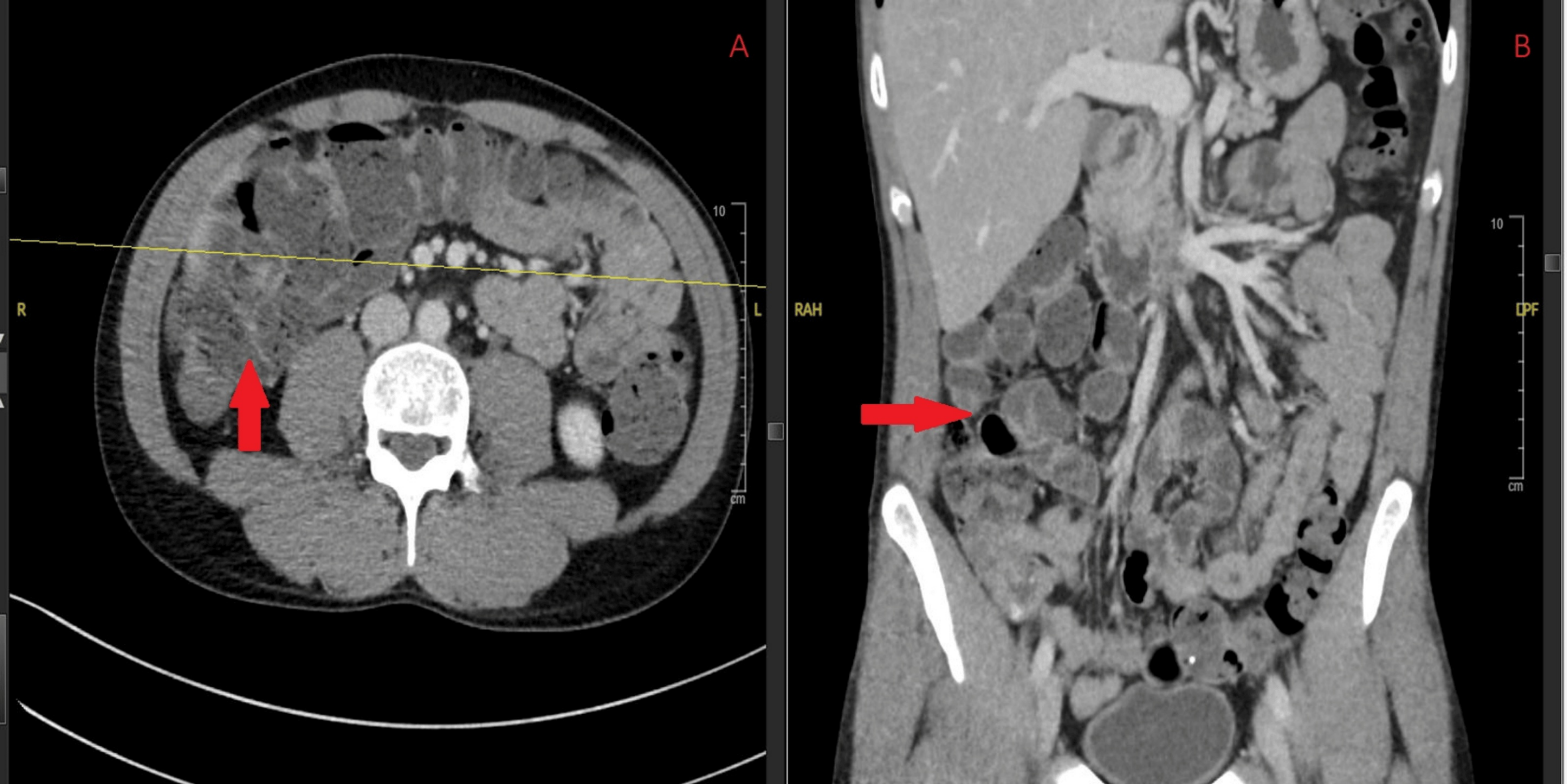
Contrast-enhanced CT of the abdomen showing right-sided colitis (arrows) with slight interval enlargement of a borderline periportal lymph node and stable subcentimeter retroperitoneal lymph nodes. (A) Axial view. (B) Coronal view. CT: computed tomography
